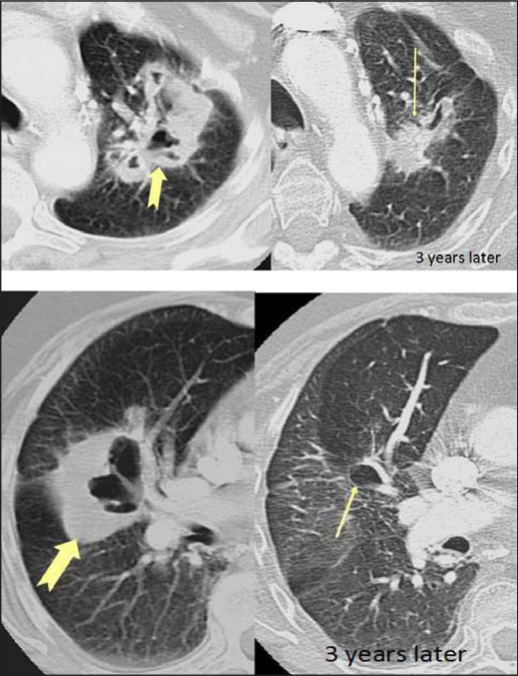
Granulomatosis with polyangiitis.* A 74-year-old female was admitted due to dyspnea and productive cough, but no fever. The CT images show relatively large cavitary lesion in both lungs with thick, irregular walls (thick arrows). Three years later, the nodules had evolved into a cyst on the right side. On the left side, the nodule was smaller and no longer cavitary.
